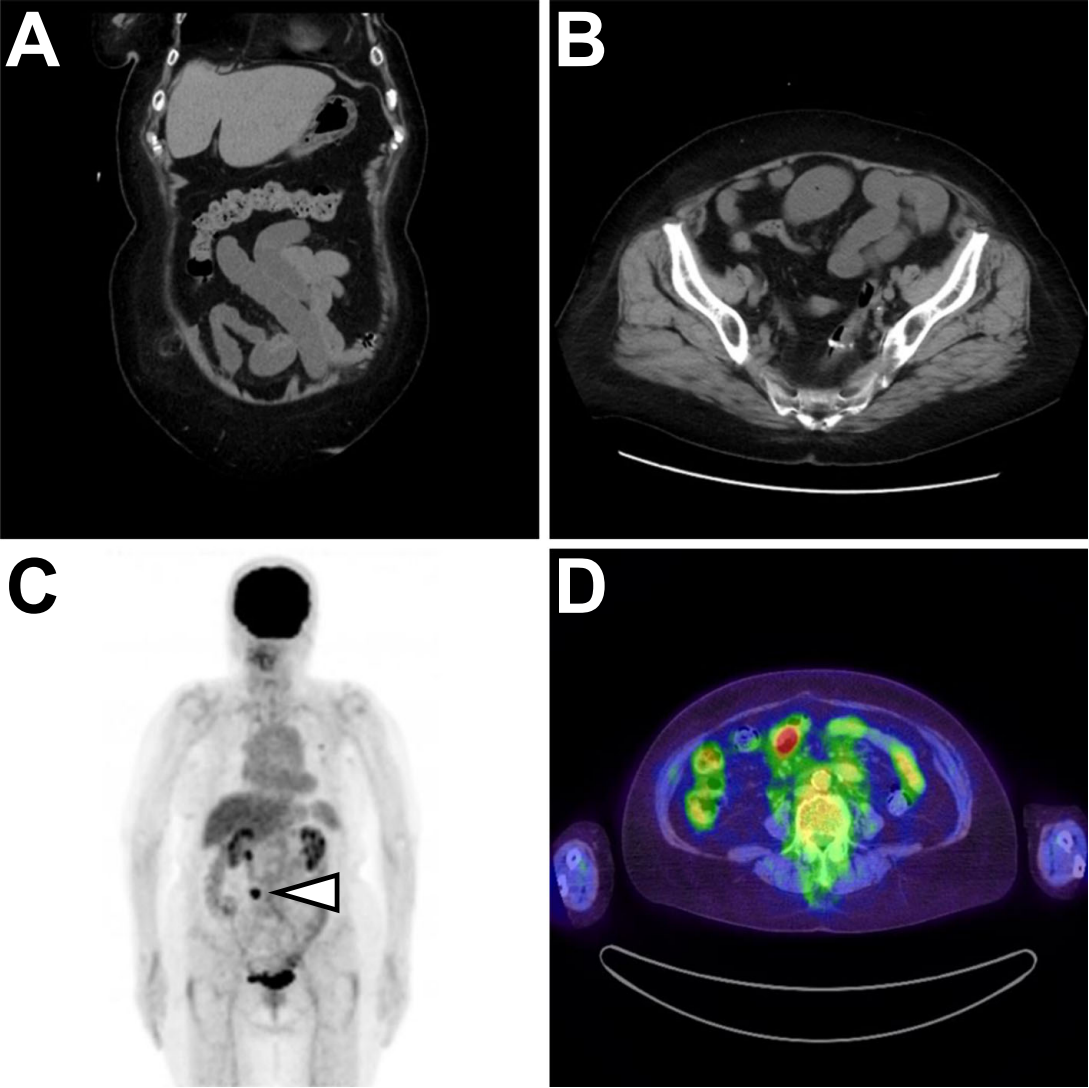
Radiological findings of the small intestinal tumor. a Coronal and b axial sections of the computed tomography scan. c Fluorodeoxyglucose positron emission tomographic scan showing an abdominal nodule (arrowhead). d The nodule is located on the small intestinal wall. The maximum standardized uptake value of the nodule was 12.81
